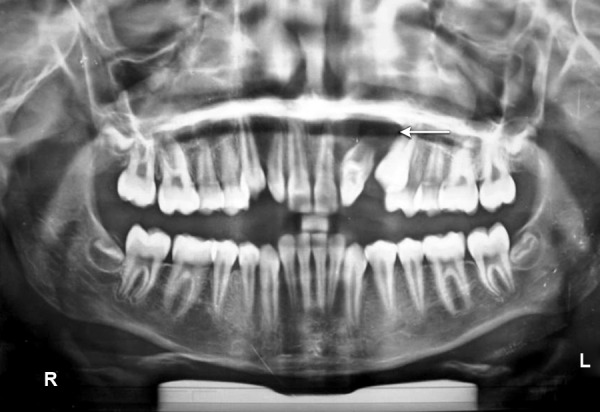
Panoramic radiograph showing a dens invaginatus in relation to 22 associated with a periapical radiolucency surrounded by a sclerotic border (arrow)
